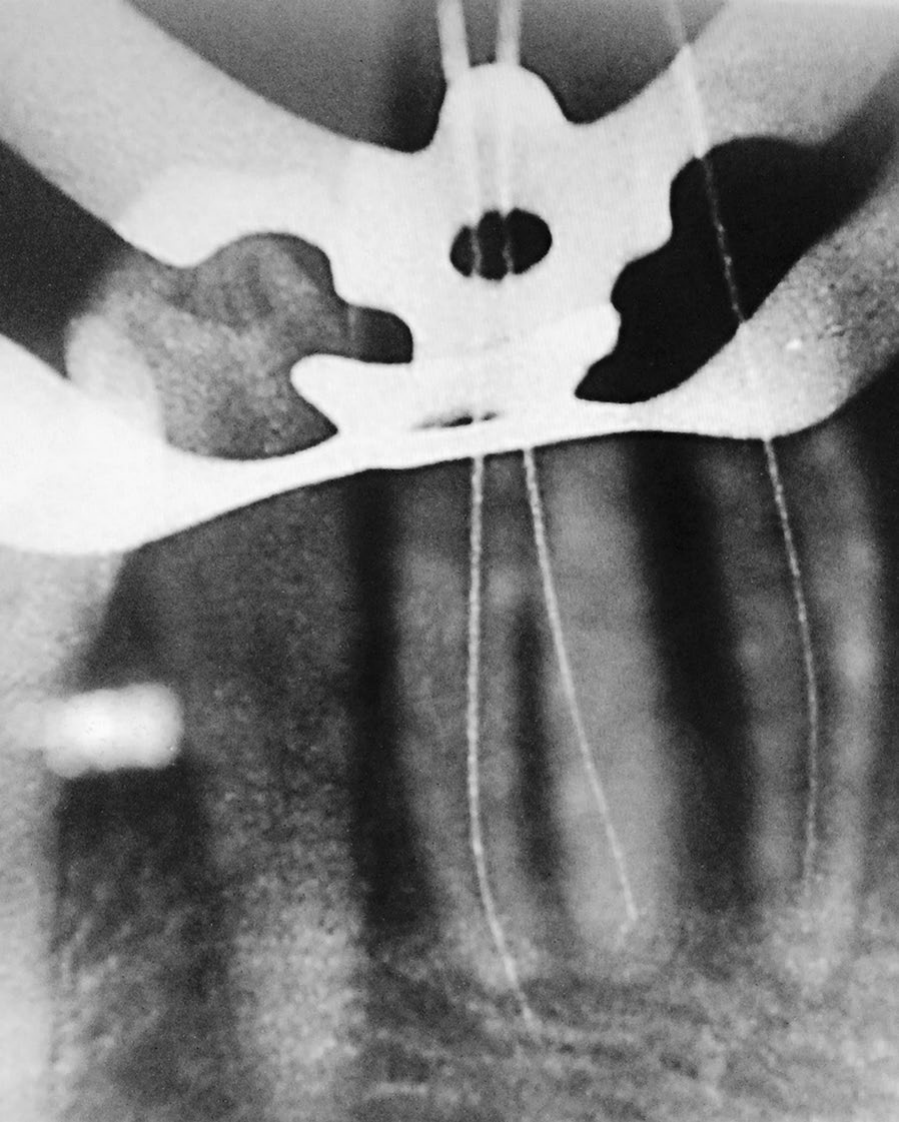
Test file radiograph to determine the working length
